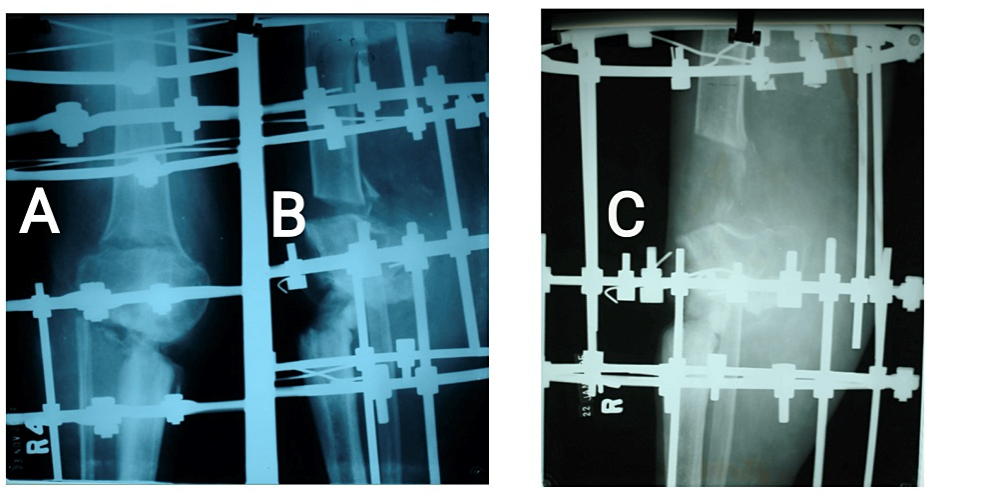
Sequential X-rays showing initial distraction followed by completed lengthening at the corticotomy site. (A) Anteroposterior view; (B) Lateral view both after initial distraction (one week); (C) Lateral view of X-ray after complete lengthening (after 10 weeks).
